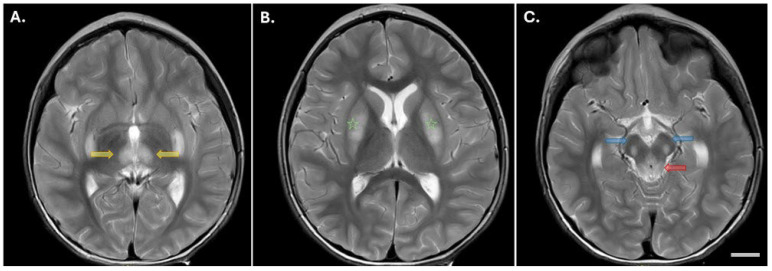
MRI findings in patient 1. (A–C) Brain MRI T2 axial section showing abnormal symmetric signals within the medial portion of the thalamus (yellow arrows); (B) within both the caudate and putamen (green star), and (C) within the substantia nigra (blue arrows) and periaqueductal gray matter (red arrow). Scale bar = 15 mm.
